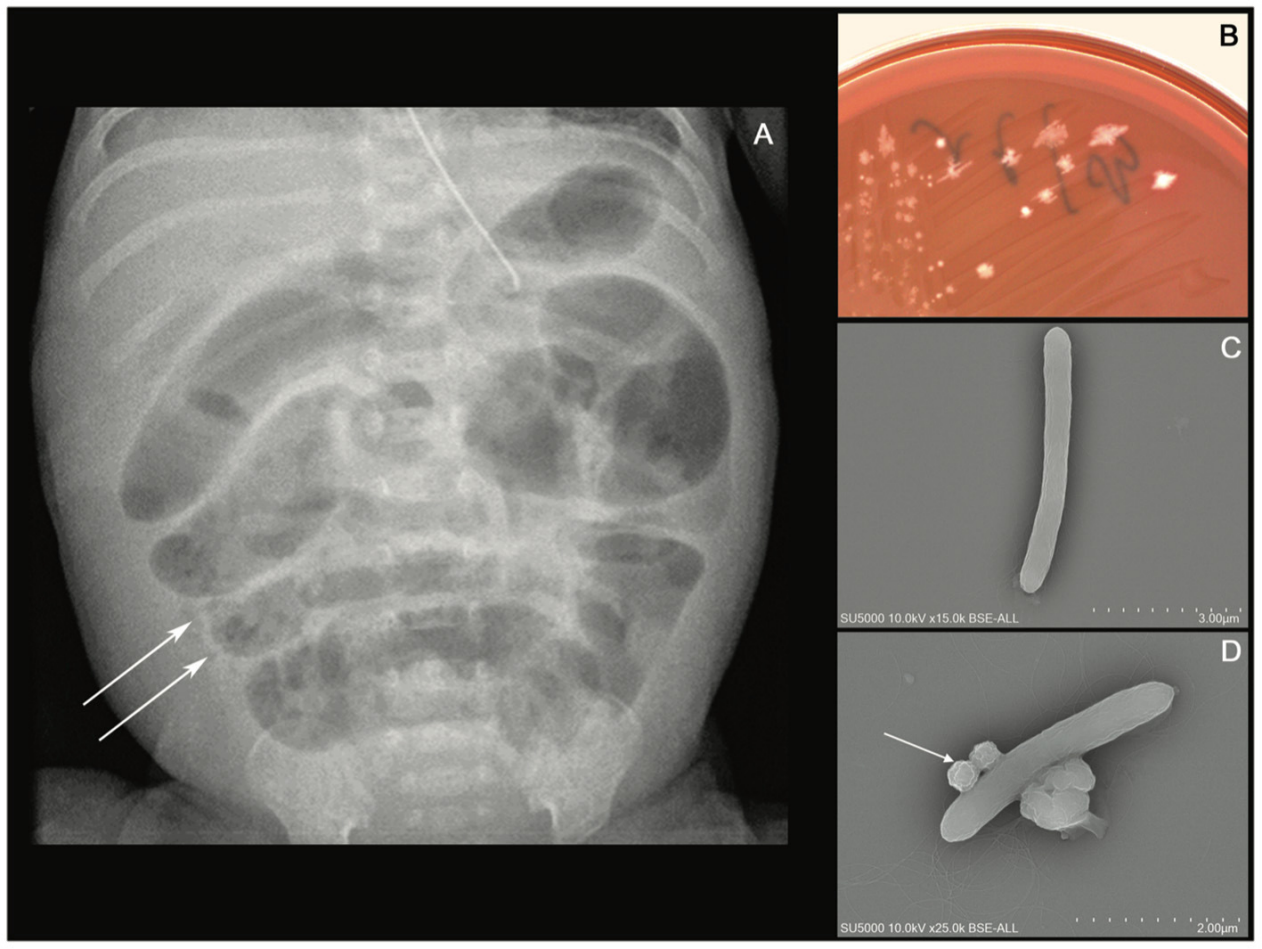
(A) Abdominal X-ray showing intramural bowel gas also known as *pneumatosis intestinalis*, (B) culture growth of cream-colored colonies on COS after 24 h of incubation at 37°C in the anaerobic chamber, (C)
